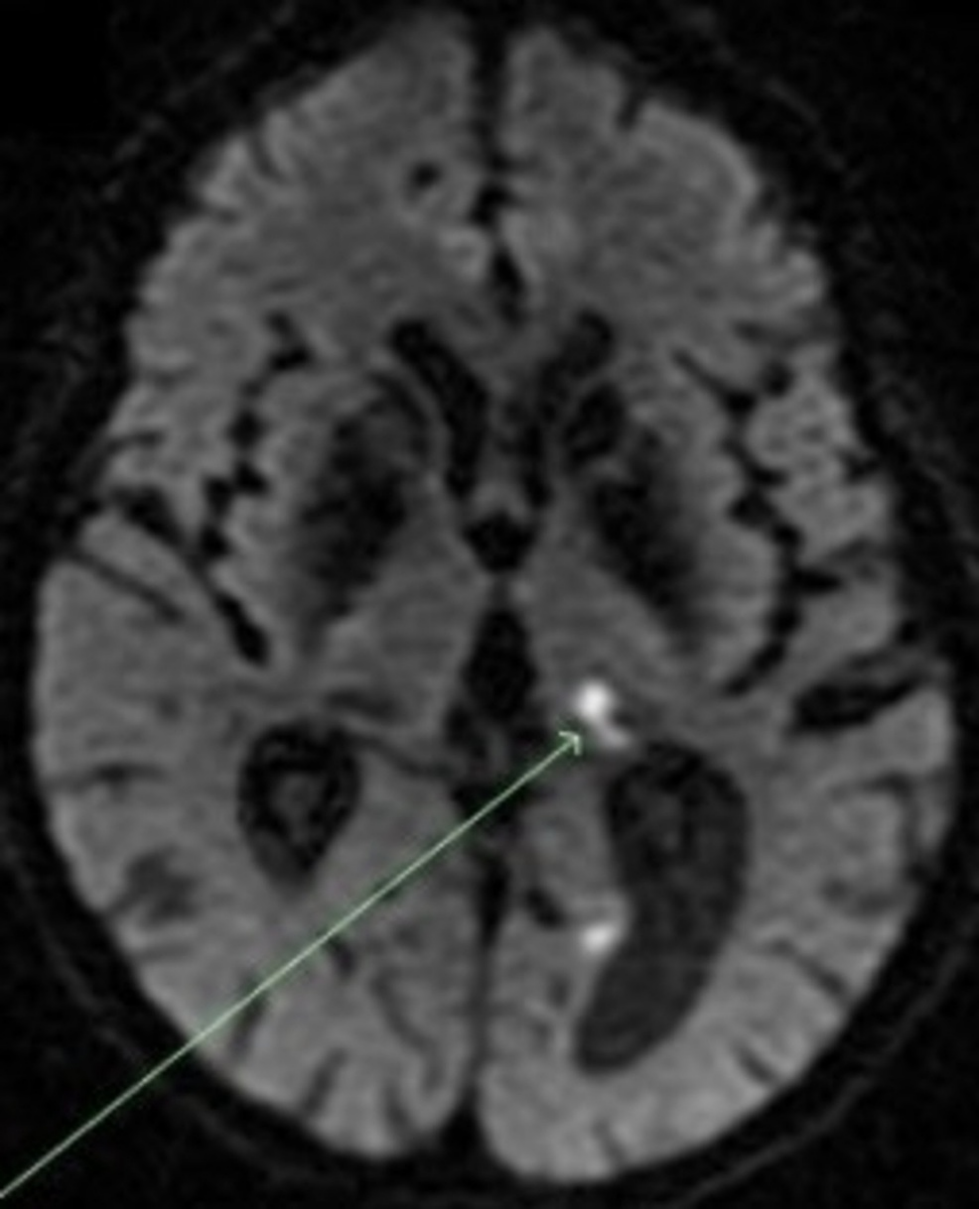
DWI sequence, axial view This image depicts an axial view of a DWI sequence that shows restricted diffusion in the left occipital lobe and in the medial left thalamus (indicated by the green arrow).
